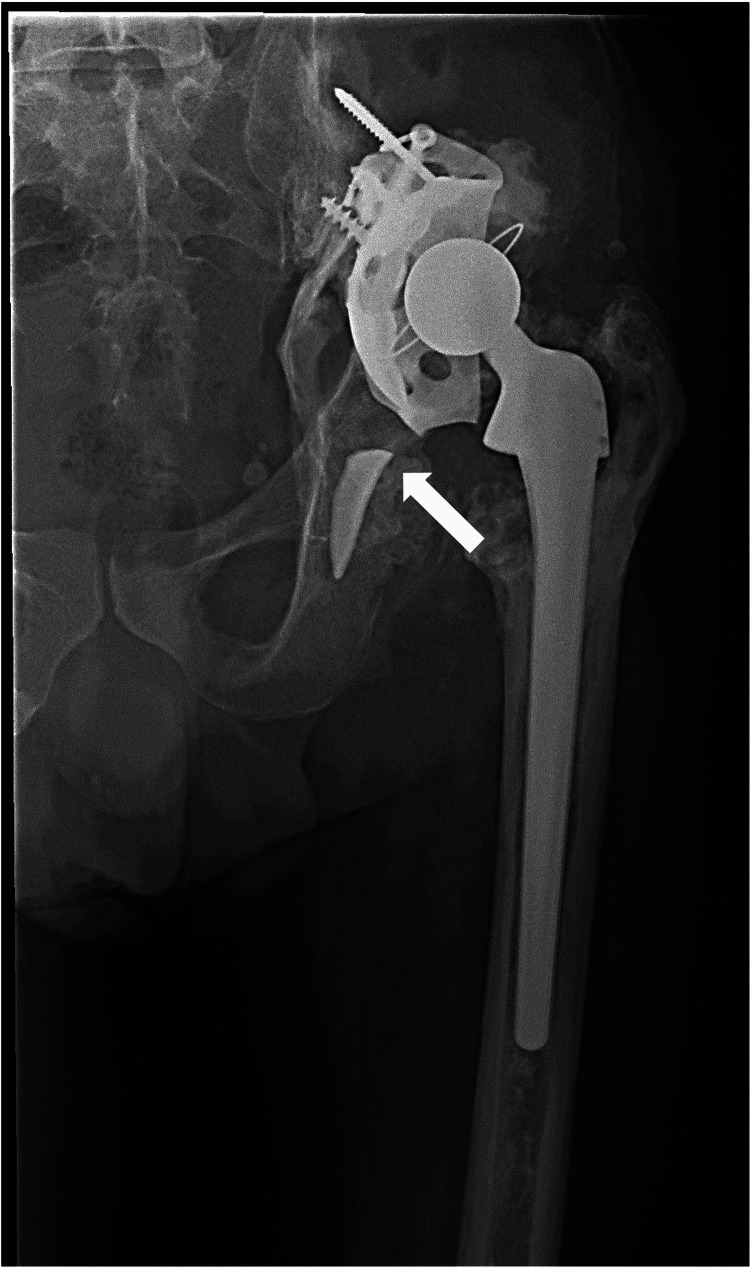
Radiograph findings (2014) In 2014, five years after acetabular revision, imaging showed a fracture of the distal cage flange (white arrow), with lateralization and verticalization of the cage.
